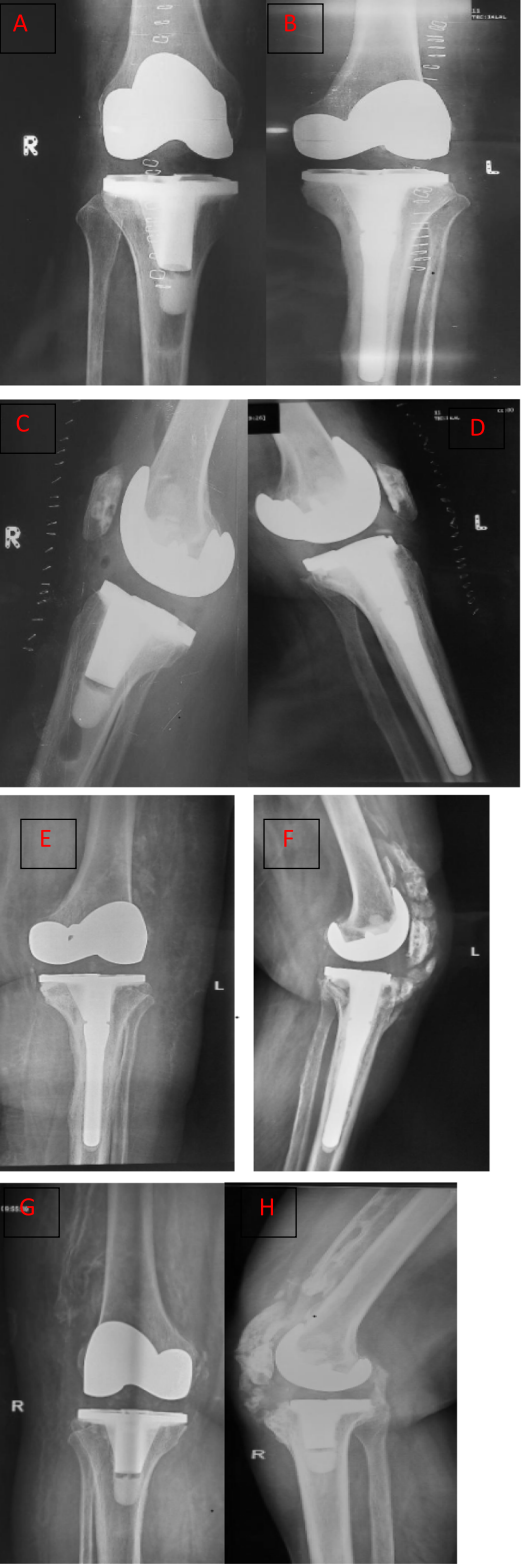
Postoperative AP view of right knee showing the result of cemented total knee arthroplasty (TKA). b AP view of left knee showing the result of cemented TKA. c. Lateral view of right knee. d Lateral view of left knee. e AP X-ray showing HO on the lateral aspect of distal femur 3 months after TKA. f Lateral view showing HO extending from the tibial tubercle to the proximal tibia. g Postoperative AP view of right knee showing HO on the lateral aspect of distal femur. h Lateral view of right knee showing severe HO on the anterior aspect of distal femur
